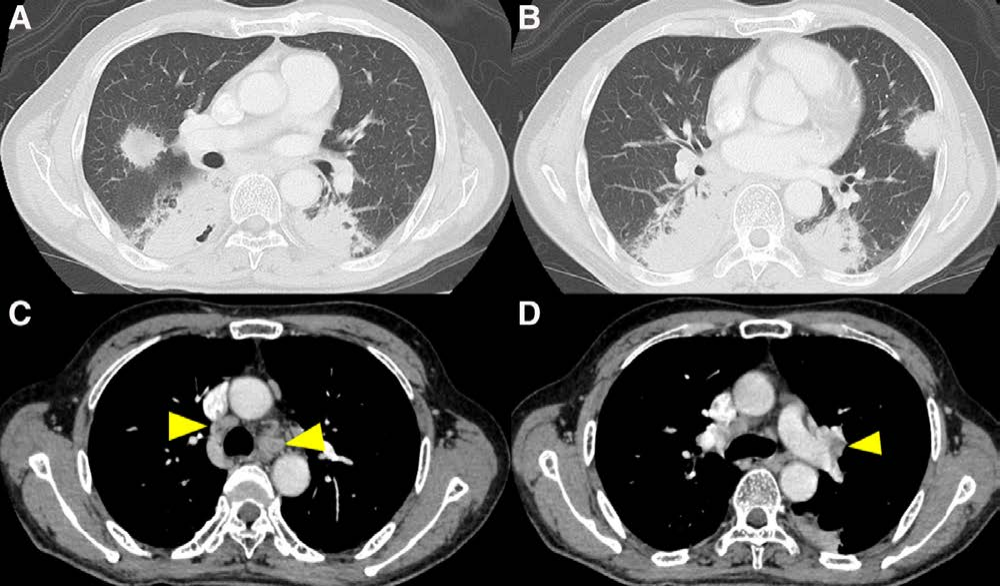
(A and B) Neck-to-pelvis CT revealing bilateral infiltrative shadows in the lower lobes of both lungs, as well as mass shadows in the both lungs. (C and D) Swelling of the bilateral hilar (arrowhead) and mediastinal (arrowhead) lymph nodes. CT = computed tomography.
